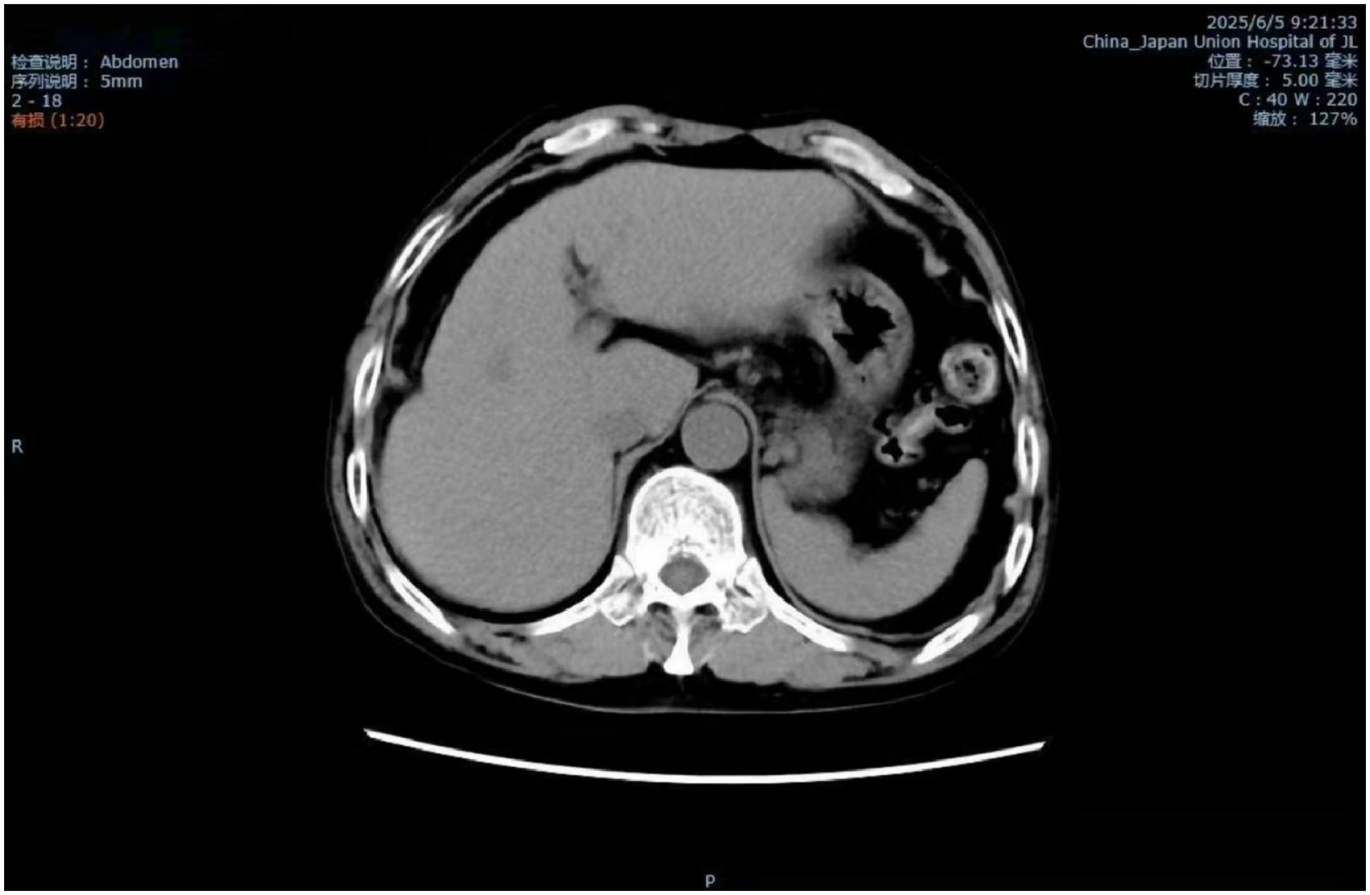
CT scan of the whole abdomen.
